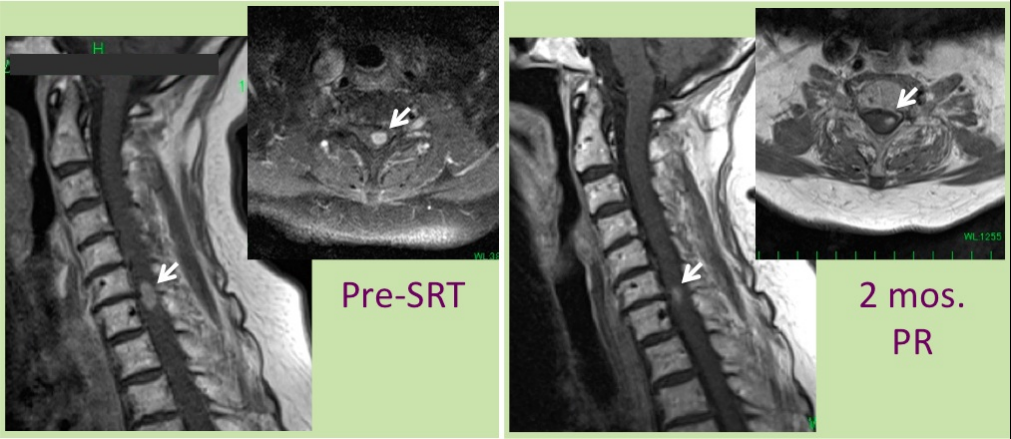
Pre-SRT and post-SRT MRIs of cervical spinal lesion *Left *: sagittal and axial view of gadolinium (Gd) enhanced magnetic resonance images (MRI) before stereotactic radiotherapy (SRT). *Right*: sagittal and axial view two months after SRT. A spinal intramedullary lesion (arrows) at the level of cervical (C) 6th and 7th vertebra shrank within two months after volumetric arc modulated therapy (VMAT) SRT.
